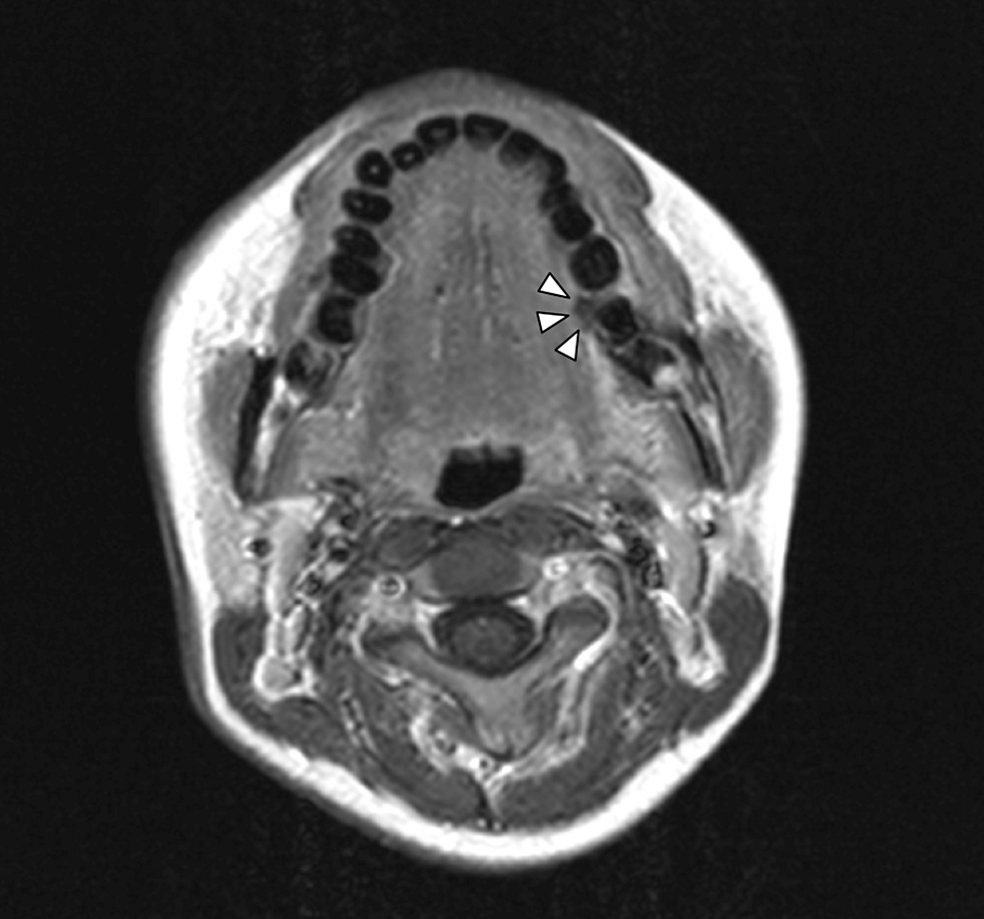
MRI image Contrast-enhanced T1-weighted MRI shows a mass with a slight contrast effect in the lesion area (white arrowheads).
